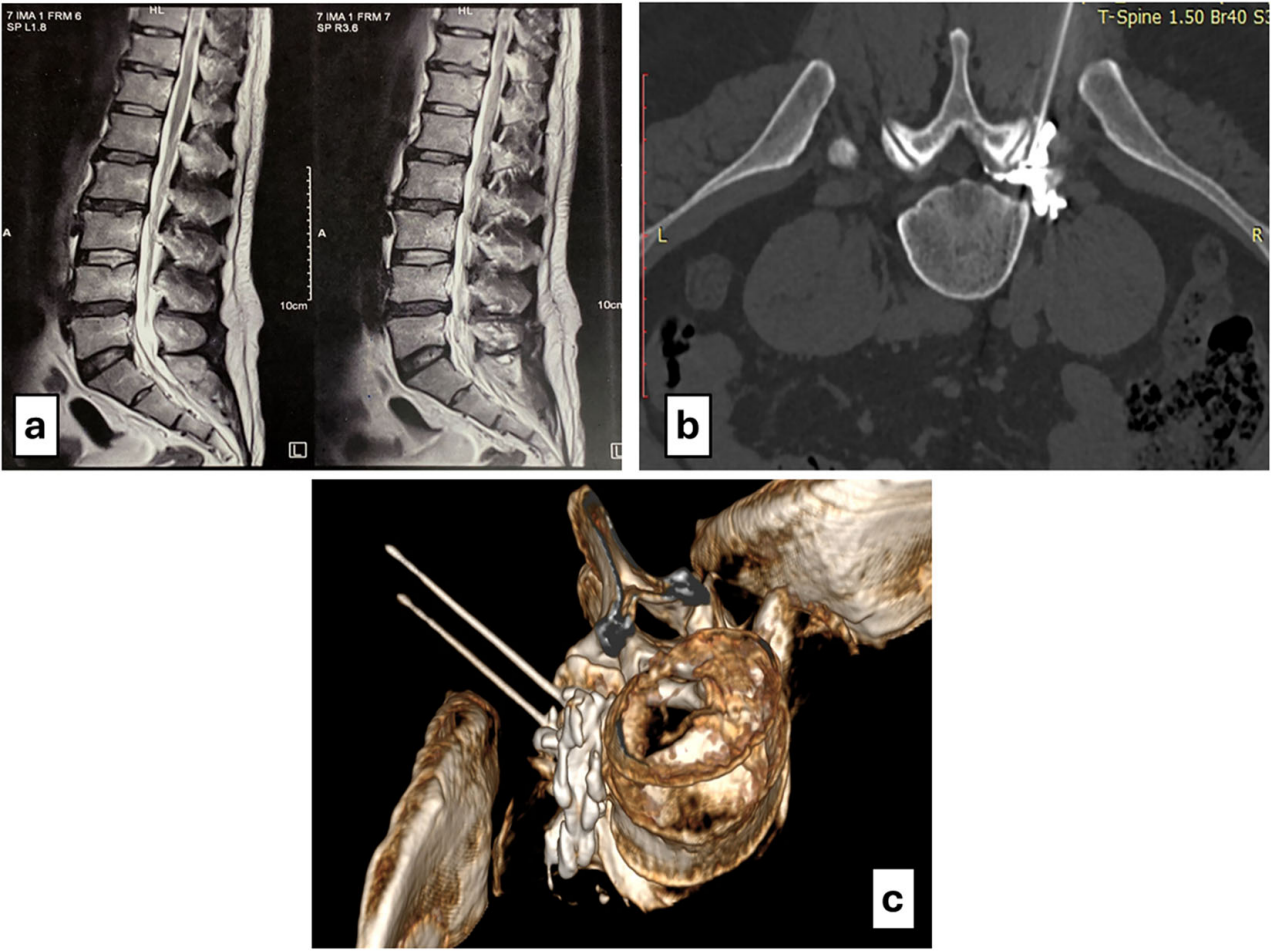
a Sagittal T2-weighted MRI of the lumbosacral spine in a 40-year-old male with diffuse posterior disc bulge at the L4–L5 and L5–S1 levels. Clinical examination revealed radicular pain in the L4 and L5 dermatomes. b Axial CT image demonstrating needle placement and contrast medium distribution at the right L4/L5 level. c 3D CT reconstruction showing RF needle placement at the foraminal positions of the right L4 and L5 nerve roots with contrast tracking
